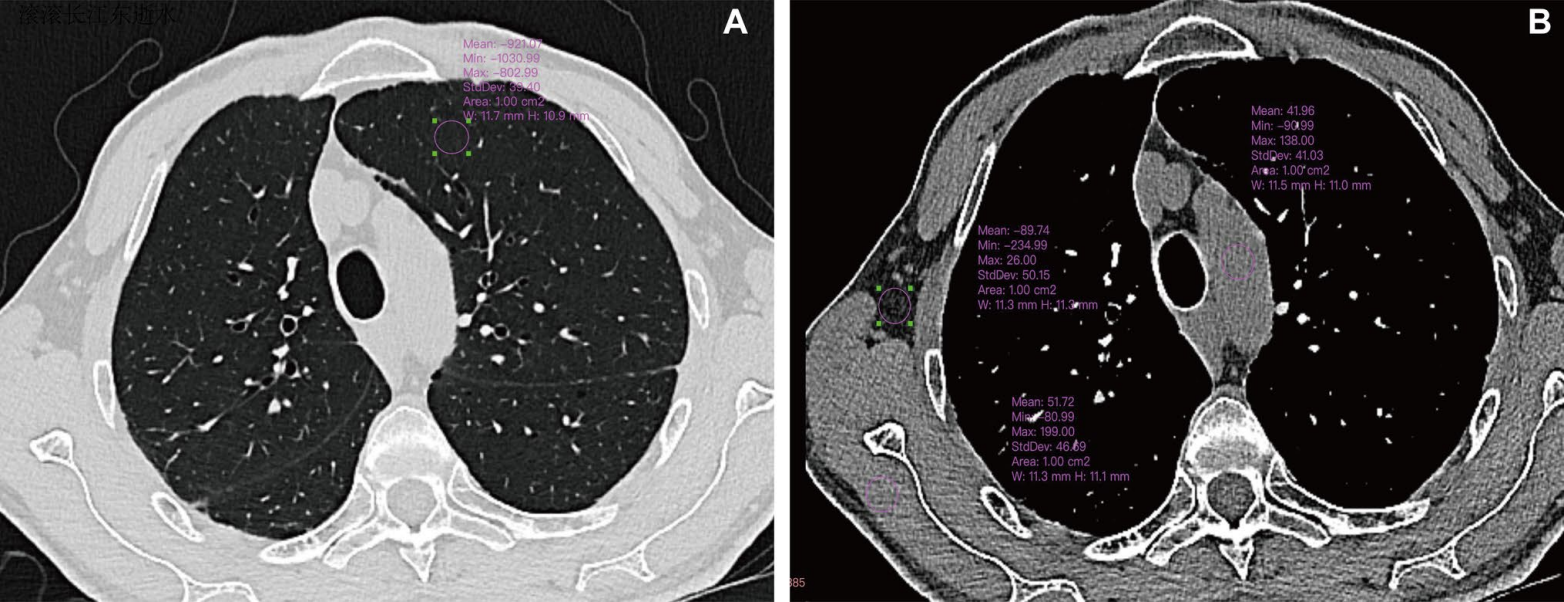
An example of image quality assessment. Signal-to-noise ratio (SNR) = ∣CT _lung_ = (-921HU) / SD _lung_ = (39HU) ∣ = 23.6, Contrast-to-noise ratio (CNR) = ∣ (CT _artery_ = (42HU) – CT _muscle_ = (52HU)) / SD _fat_ = (50HU) ∣ = 0.2
